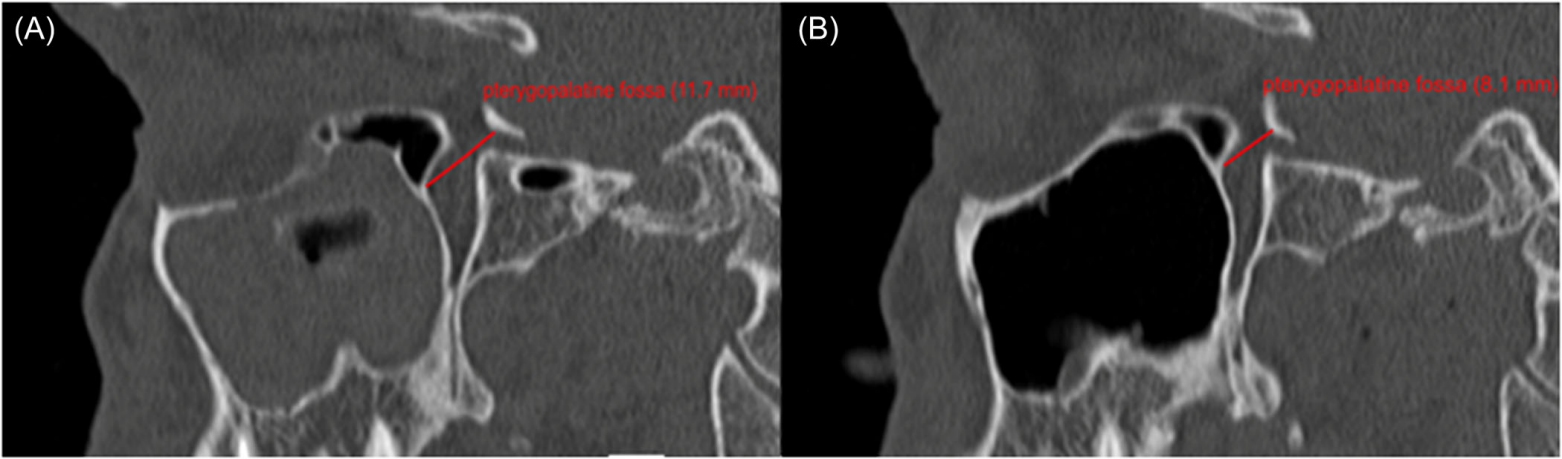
Sagittal skull CT scan of a patient suffering from SSS, (A) pterygopalatine fossa width of the SSS‐affected side (right maxillary sinus), (B) pterygopalatine fossa width of the healthy side (left maxillary sinus). CT, computed tomography; SSS, silent sinus syndrome.
